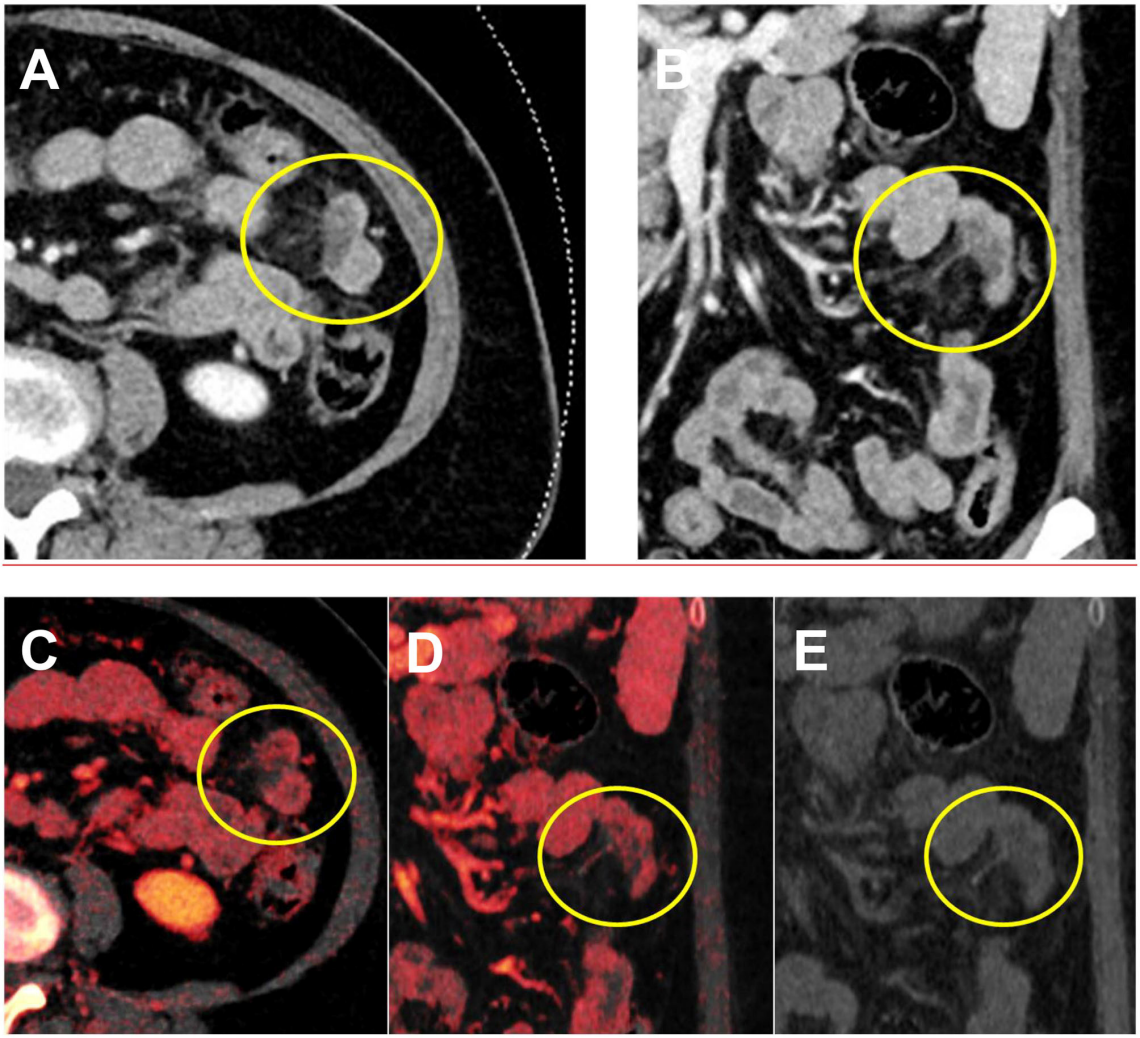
A 17-year-old male patient presenting with pain and vomiting after trauma during a basketball game. (A,B) Conventional CT demonstrates hypodensity in the bowel wall. (C,D) No internal iodine enhancement and uptake were confirmed using the iodine overlay map, confirming bowel wall hematoma. (E) On the virtual non-contrast image, the bowel wall remains hypodense.
